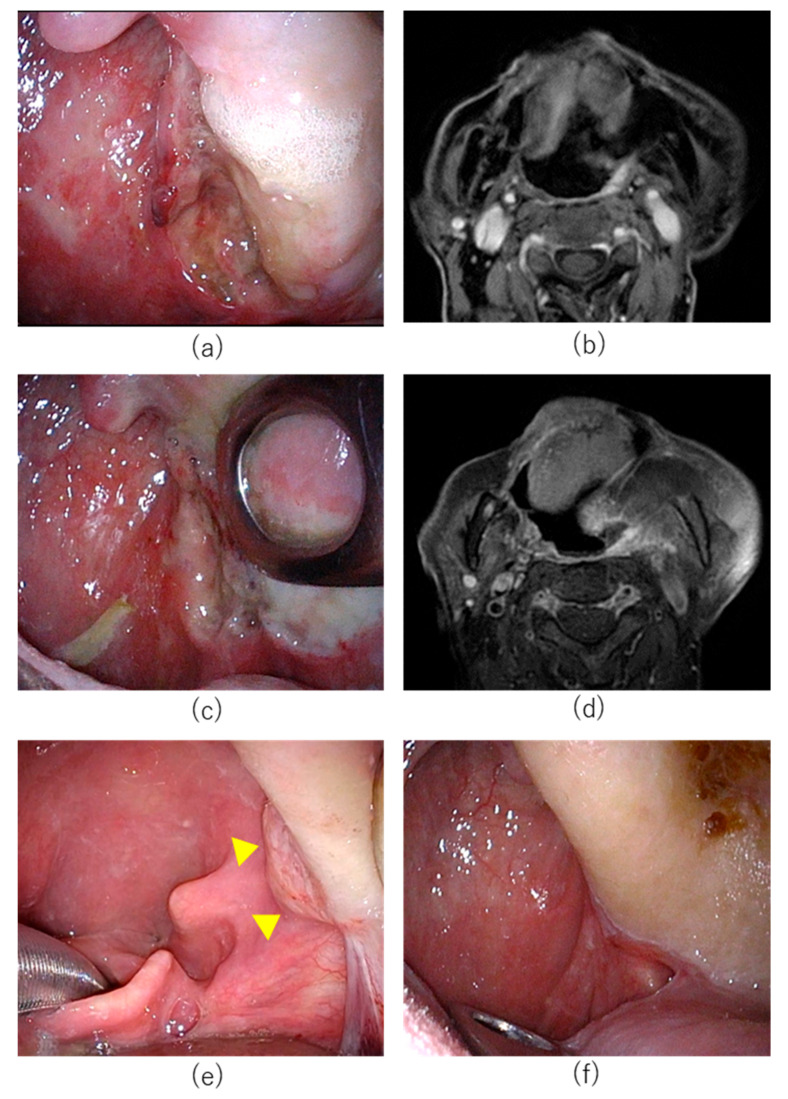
Posttreatment change after HN-PIT for Case 1. (a) Endoscopic image on postoperative day 9 after cycle 1. Ulceration was observed in the illuminated area. (b) MRI image after cycle 1. (c) Endoscopic image on postoperative day 6 after cycle 2. (d) MRI image after cycle 2. (e) Intraoperative image of the tumor (arrowheads) before transoral resection. (f) endoscopic image on postoperative 4 months.
